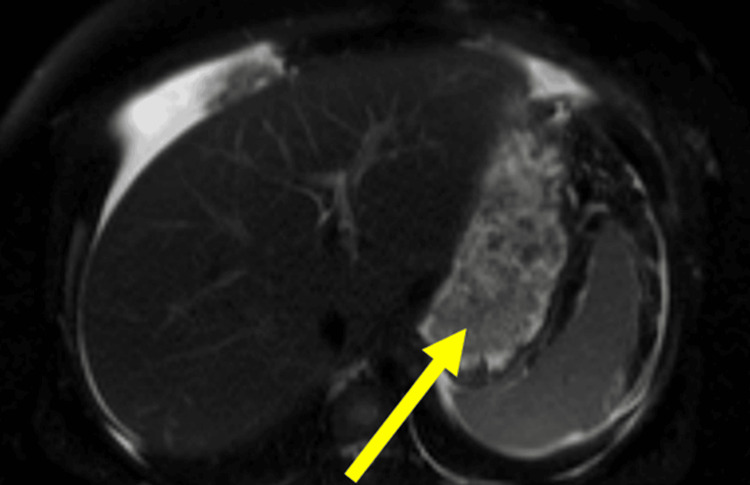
Follow-up MRI of the abdomen and pelvis showing persistent calcified intraperitoneal fluid collection (15.8×9.9×2 cm) despite aspiration and drainage of fluid collection seven days prior MRI: magnetic resonance imaging
